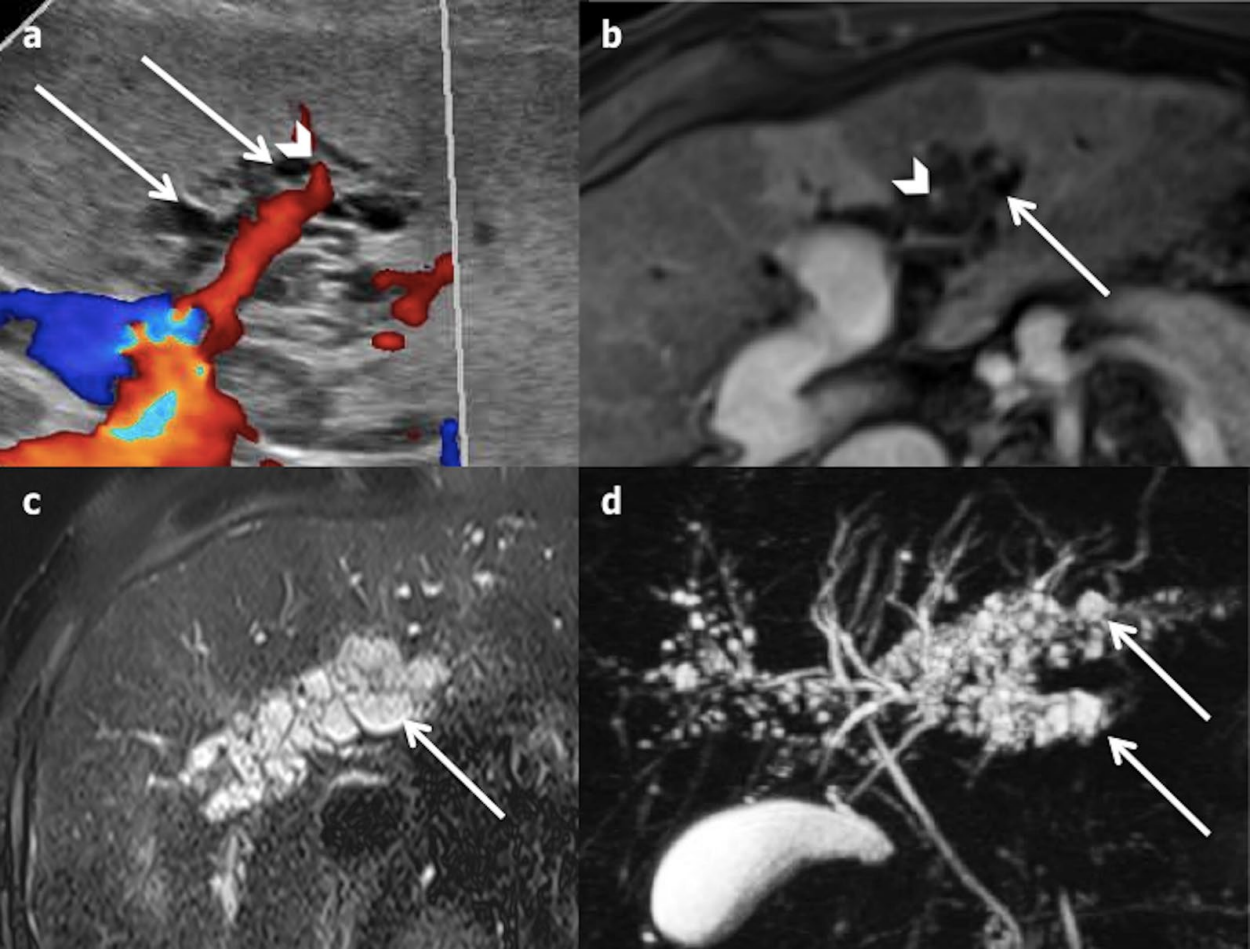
Peribiliary cyst in a 69-year-old cirrhotic male. a Ultrasonography shows multiple periportal (arrowhead) cysts (arrows). b The portal vessels are clearly visible (arrowhead) on axial portal venous phase magnetic resonance imaging, showing the periportal location of the cysts. c and d Axial T2-weighted magnetic resonance imaging and magnetic resonance cholangiopancreatography images show multiple small cysts along thin, non-dilated bile ducts
